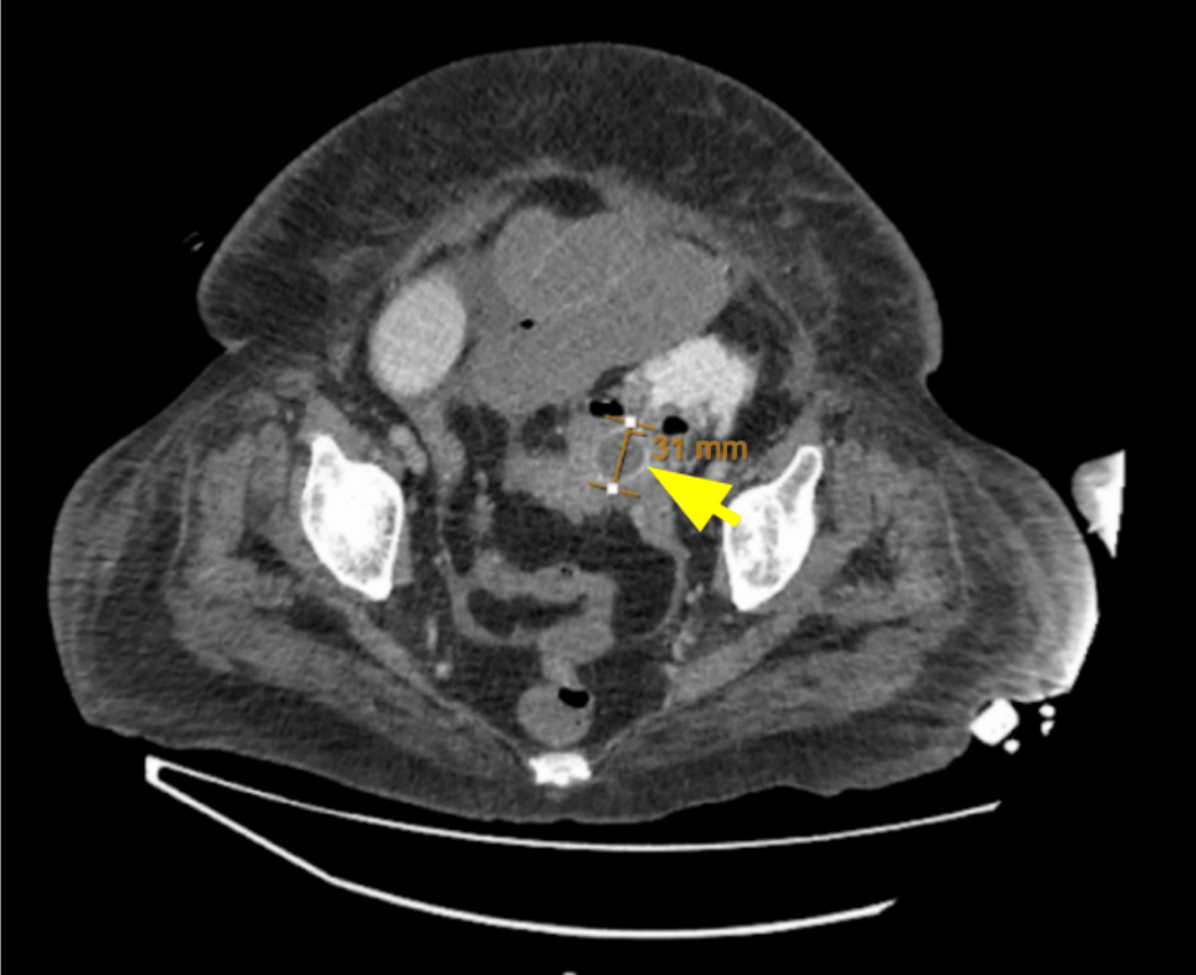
Axial CT image of the abdomen and pelvis showing an impacted 31 mm gallstone in the sigmoid colon, indicated by a yellow arrow
